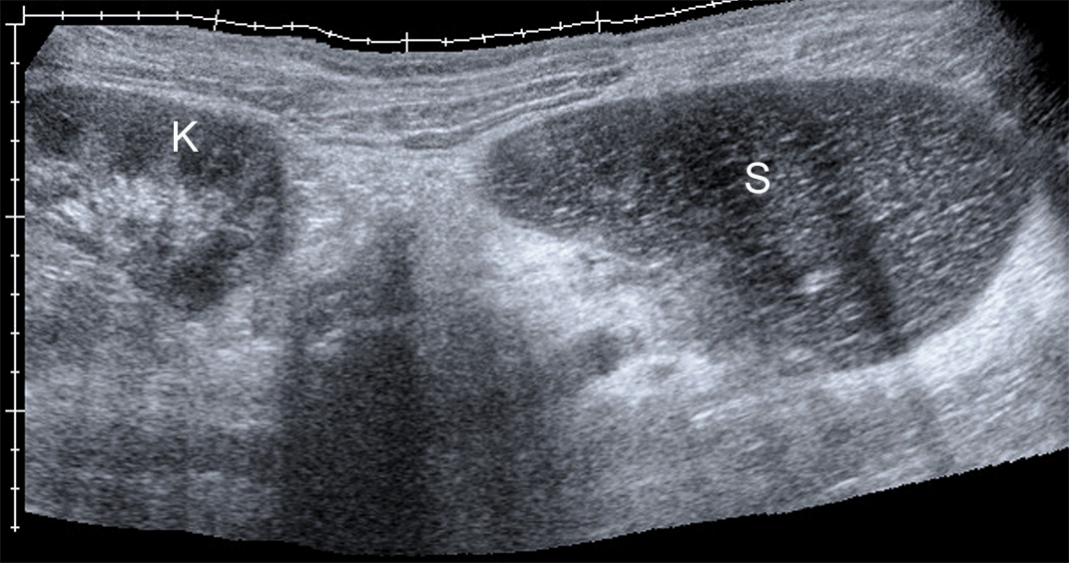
Greyscale ultrasound panorama of the left hypochondrium and flank. The spleen appears as a homogeneous hypoechoic comma-shaped mass (S); it should be noted that the spleen is located under the lower pole of the kidney (K) in the ectopic position.
